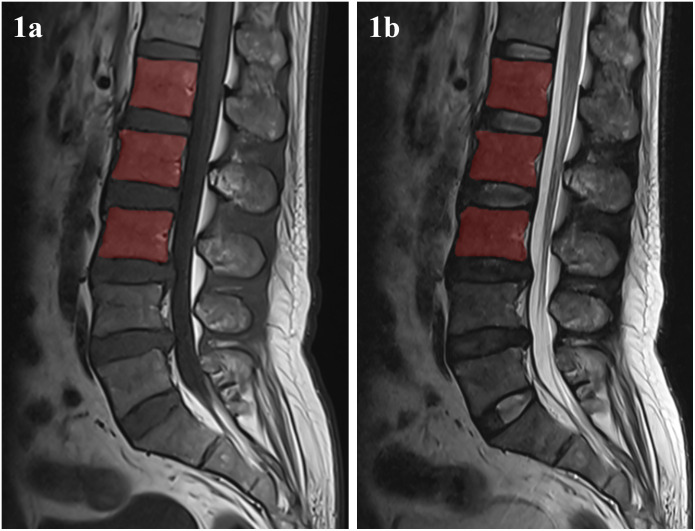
Representative volume-of-interest (VOI) in MR image of the lumbar spine. (a) and (b) are respectively the sagittal images of T1WI and T2WI.
